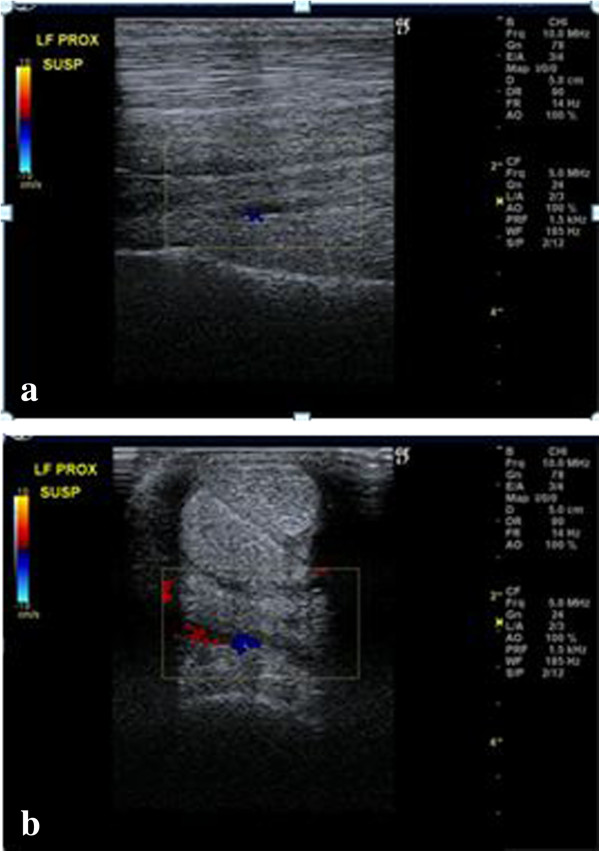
Weight-bearing a) longitudinal and b) transverse images of the proximopalmar metacarpus highlighting the deep volar arch using Doppler. Proximal is to the left on the longitudinal image and lateral is to the left on the transverse image.
